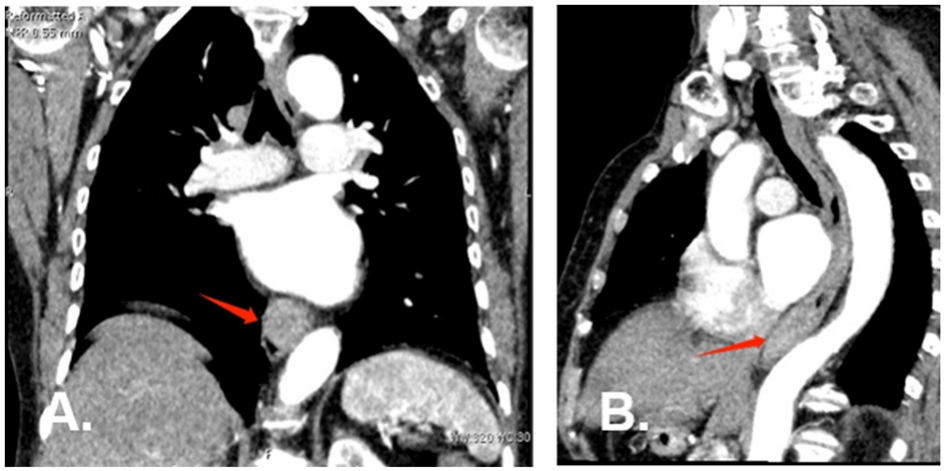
Chest contrast-enhanced computed tomography at diagnosis. (A) (Coronal plane) and (B) (Sagittal plane) CT scan revealed a well-defined homogeneous soft tissue mass at the lower esophagus (red arrow).
